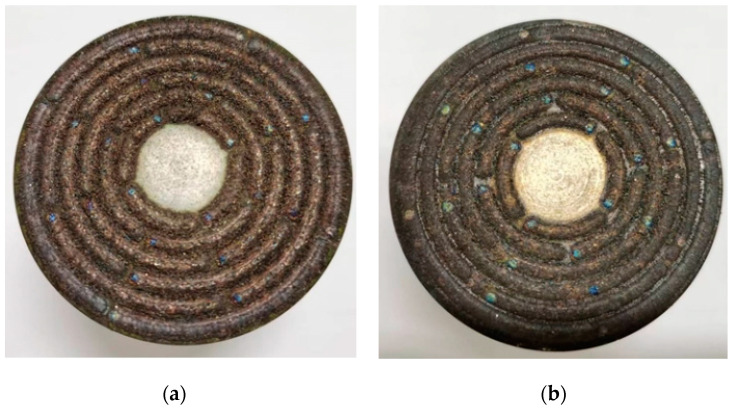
The 3D printed structured CBN grinding wheel: (a) without ultrasonic vibration; (b) with ultrasonic vibration.
